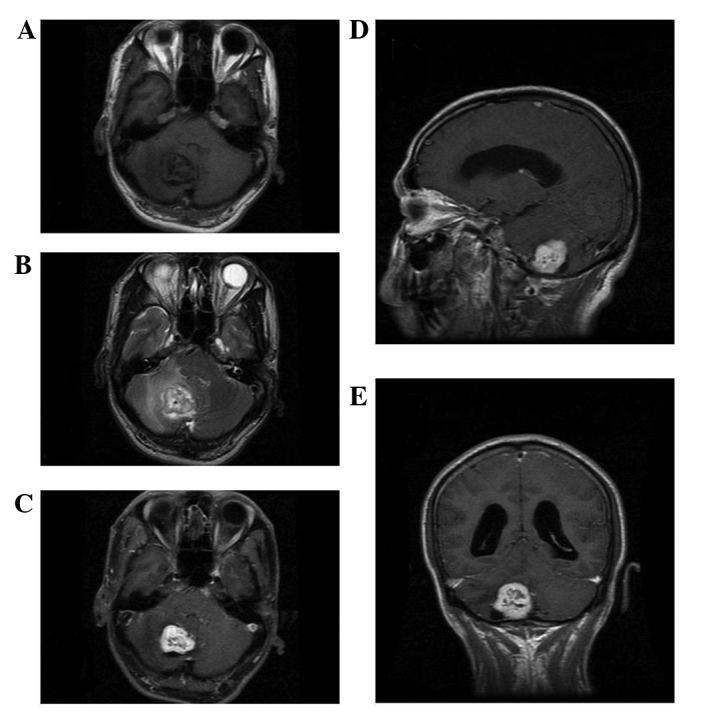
Patient 5. (A) Unenhanced magnetic resonance imaging scan reveals that the lesion is located in the infratentorial posterior fossa. (B) Peritumoral brain edema may be observed, and the fourth ventricle exhibits compressive change. (C) The lesion is markedly enhanced following injection of contrast agent. (D) The dural tail sign cannot be found, however, a (E) narrow-based attachment is visible and the intratumoral vessel may be detectable.
